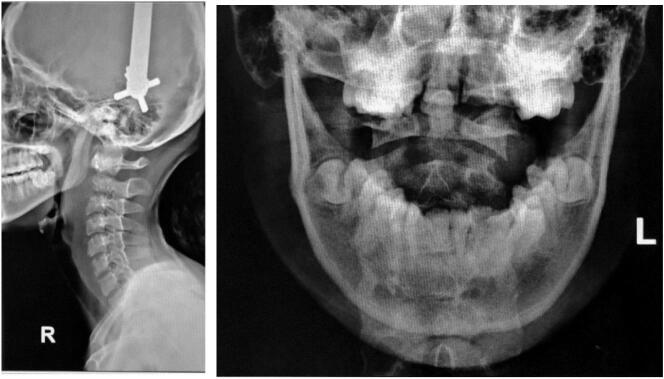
Post-traction radiographic evaluation.
